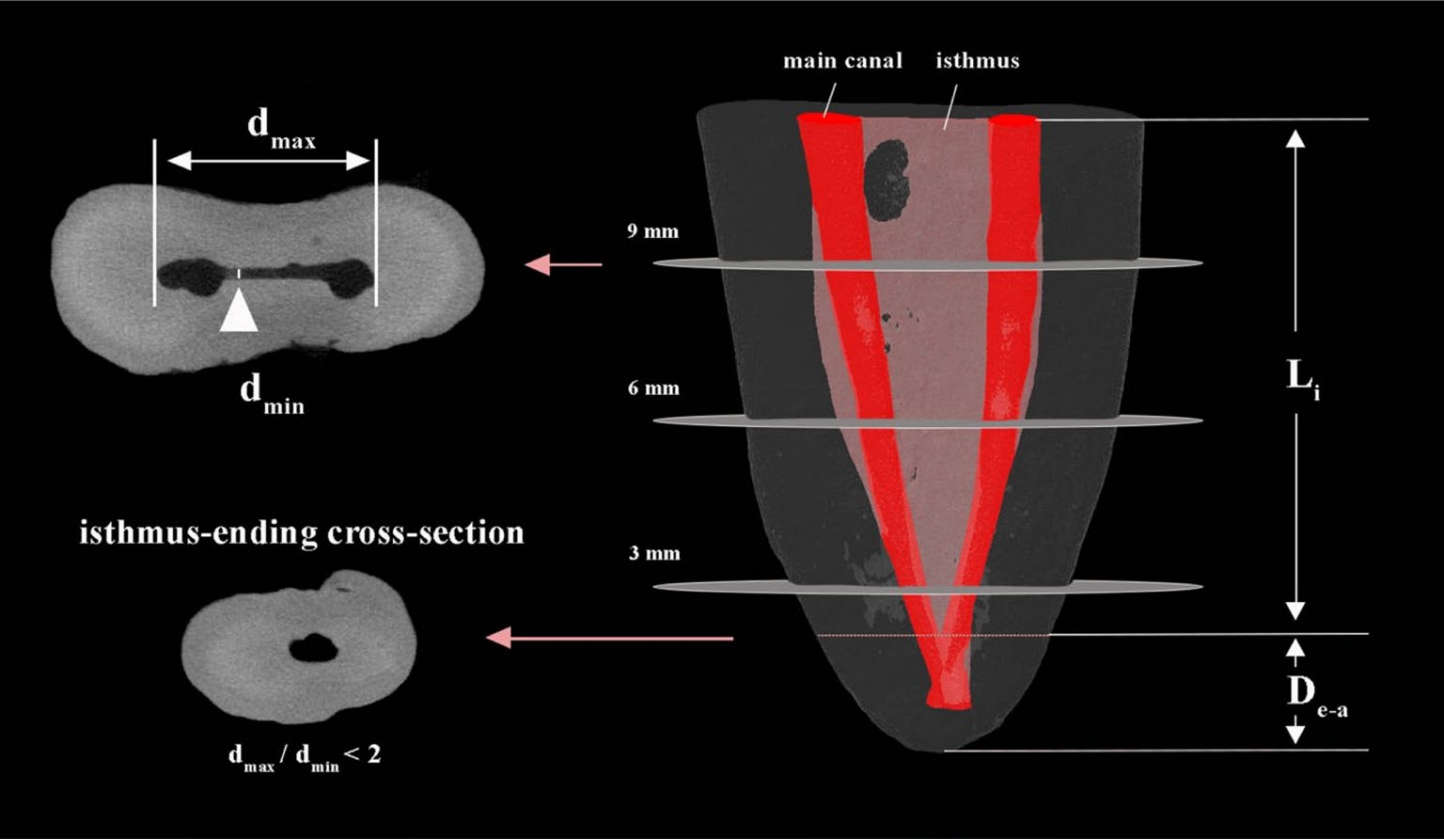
Micro‐CT analysis of isthmus morphology. The main root canals and isthmuses were digitally reconstructed in red and pink, respectively. Cross‐sectional measurements were taken at three levels: apical (3 mm from apex), middle (6 mm) and coronal (9 mm). *d*
